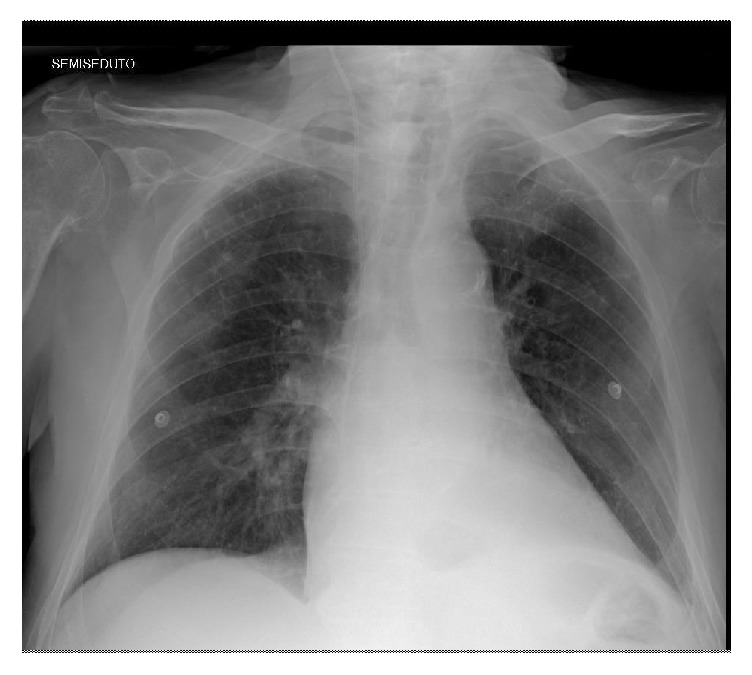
Chest radiography two months later.
